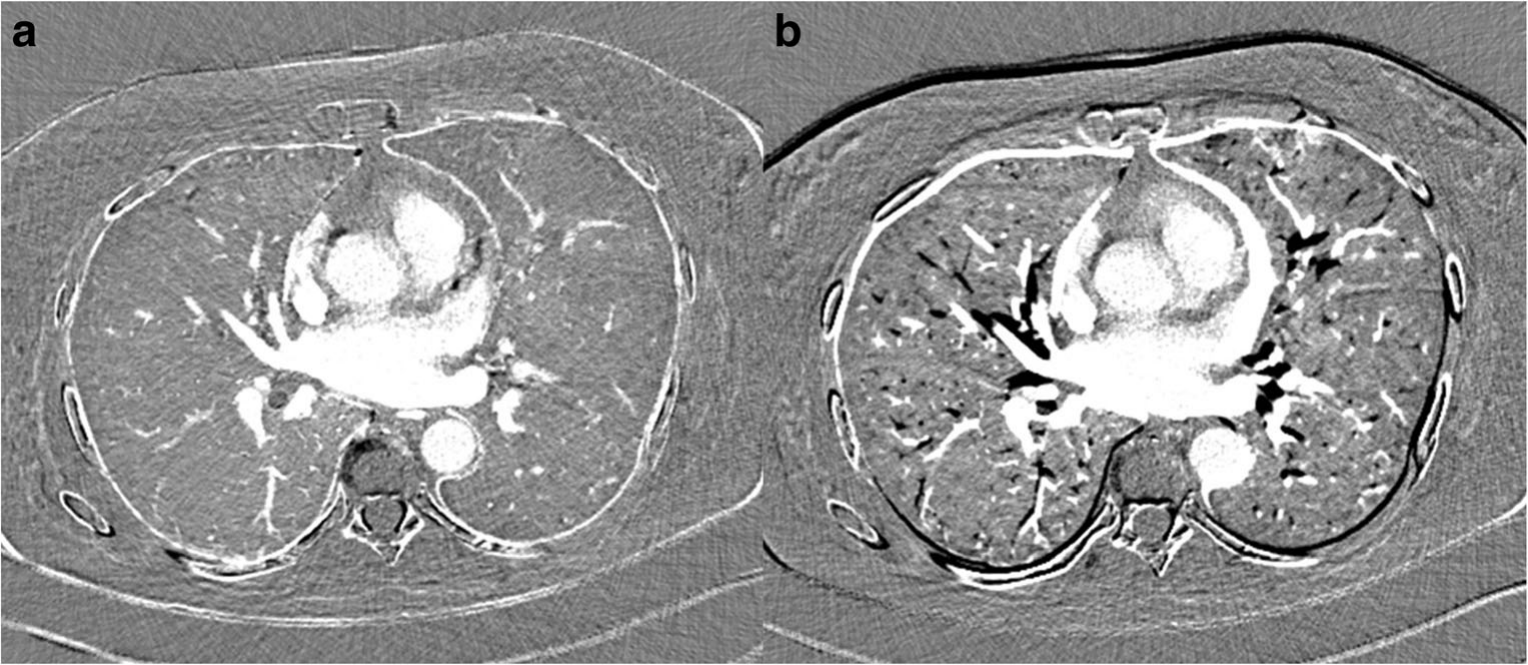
1-mm axial CTPA reconstructions after subtraction of an unenhanced CT from a CTPA with a diaphragm difference of 11 mm between the scans. a With motion correction, and (b) without motion correction
